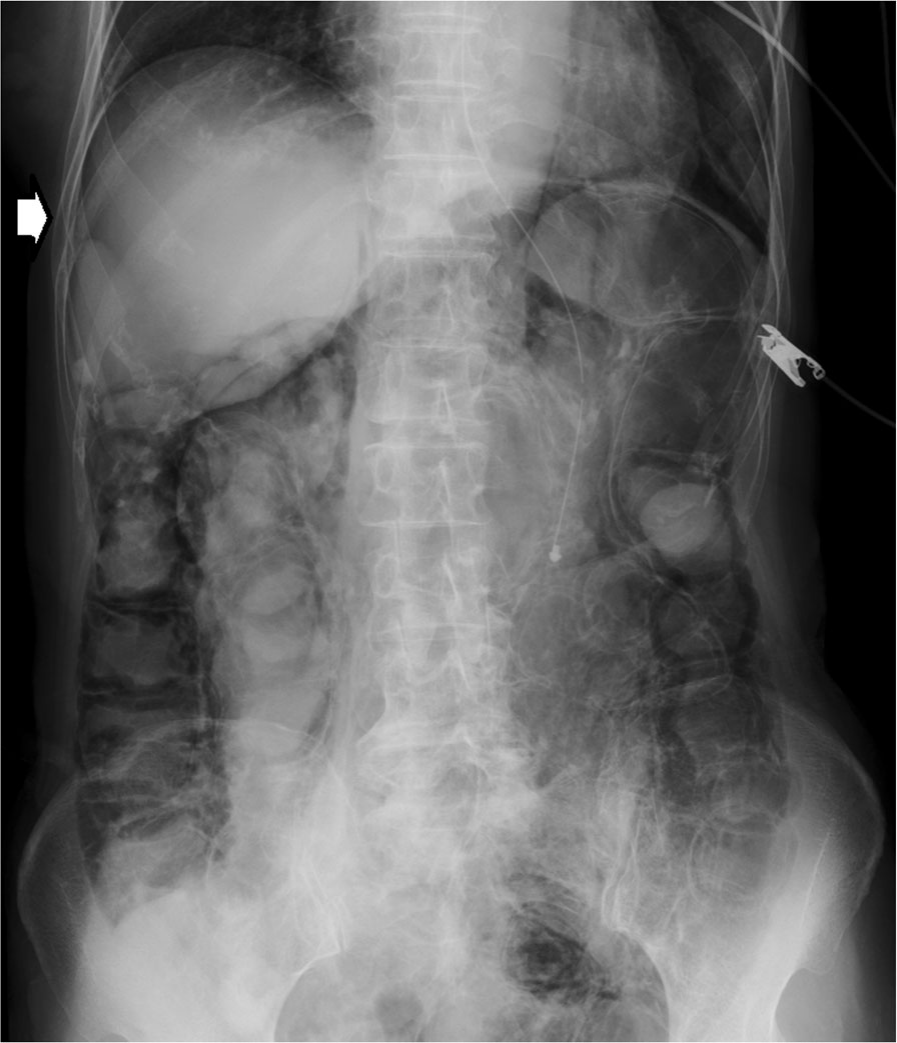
An abdominal radiograph showed a dilated colon and the presence of intraluminal air along the wall of the colon with free air (arrow)
